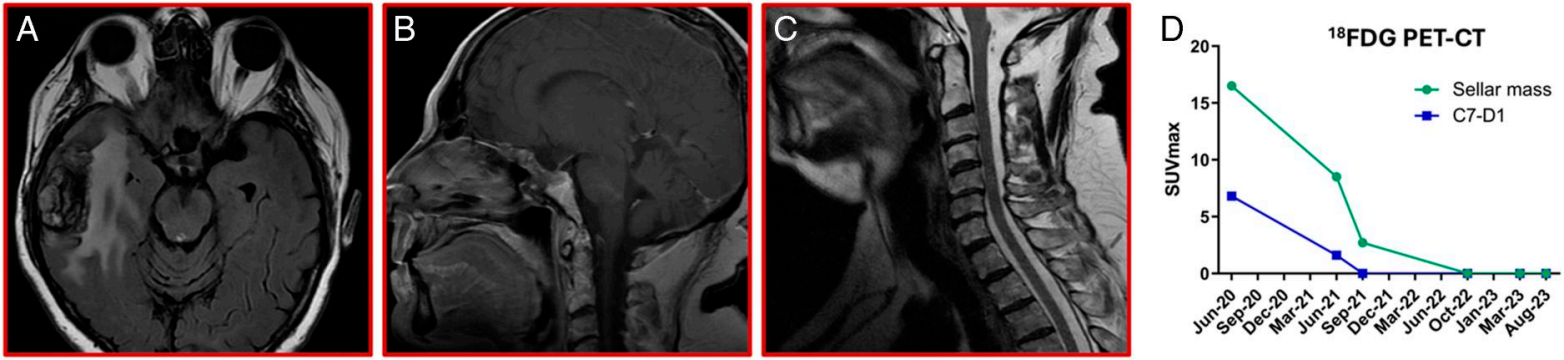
Complete radiologic and metabolic response to pembrolizumab. T1-weighted MRI performed after 21 months of treatment with pembrolizumab showing the absence of residual sellar disease—axial (A) and sagittal (B)—and the cervical spine (C). The temporal radionecrotic lesion surrounded by a noncompressive peripheral oedema was asymptomatic but already present (A). Evolution of the metabolic activity during ^18^FDG PET-CT imaging, as indicated by SUV measured on the primary sellar tumor (green line) and the spinal metastasis (blue line) (D). CT indicates computed tomography; FDG, fluorodeoxyglucose; PET, positron emission tomography; SUV, standardized uptake value. Adaptations are themselves works protected by copyright. So in order to publish this adaptation, authorization must be obtained both from the owner of the copyright in the original work and from the owner of copyright in the translation or adaptation Modified from Ref.^7^
